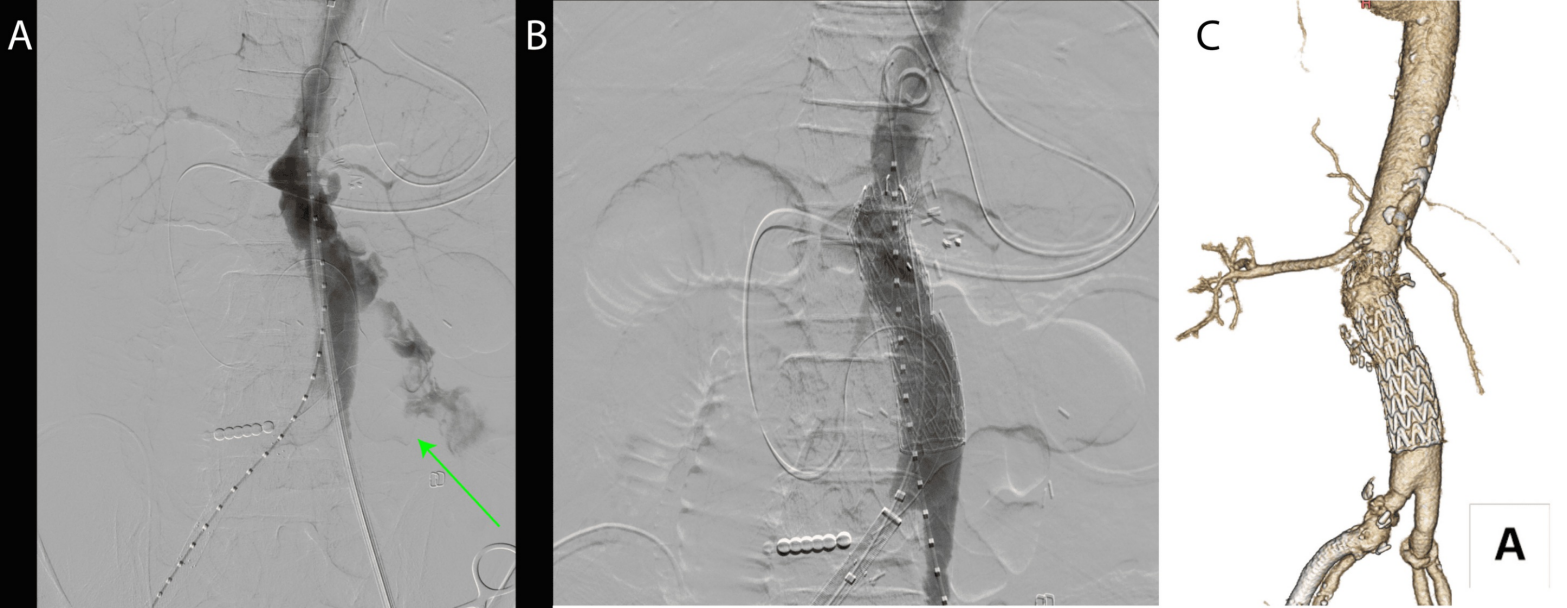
Intraoperative images during endovascular treatment of central hemorrhage (A) Bleeding from the central side of the abdominal aortic prosthesis anastomosis was observed. (green arrow); (B) Stent grafts provided hemostasis, and no bleeding was observed. (C) Enhanced computed tomography. A stent graft covers the central anastomosis.
